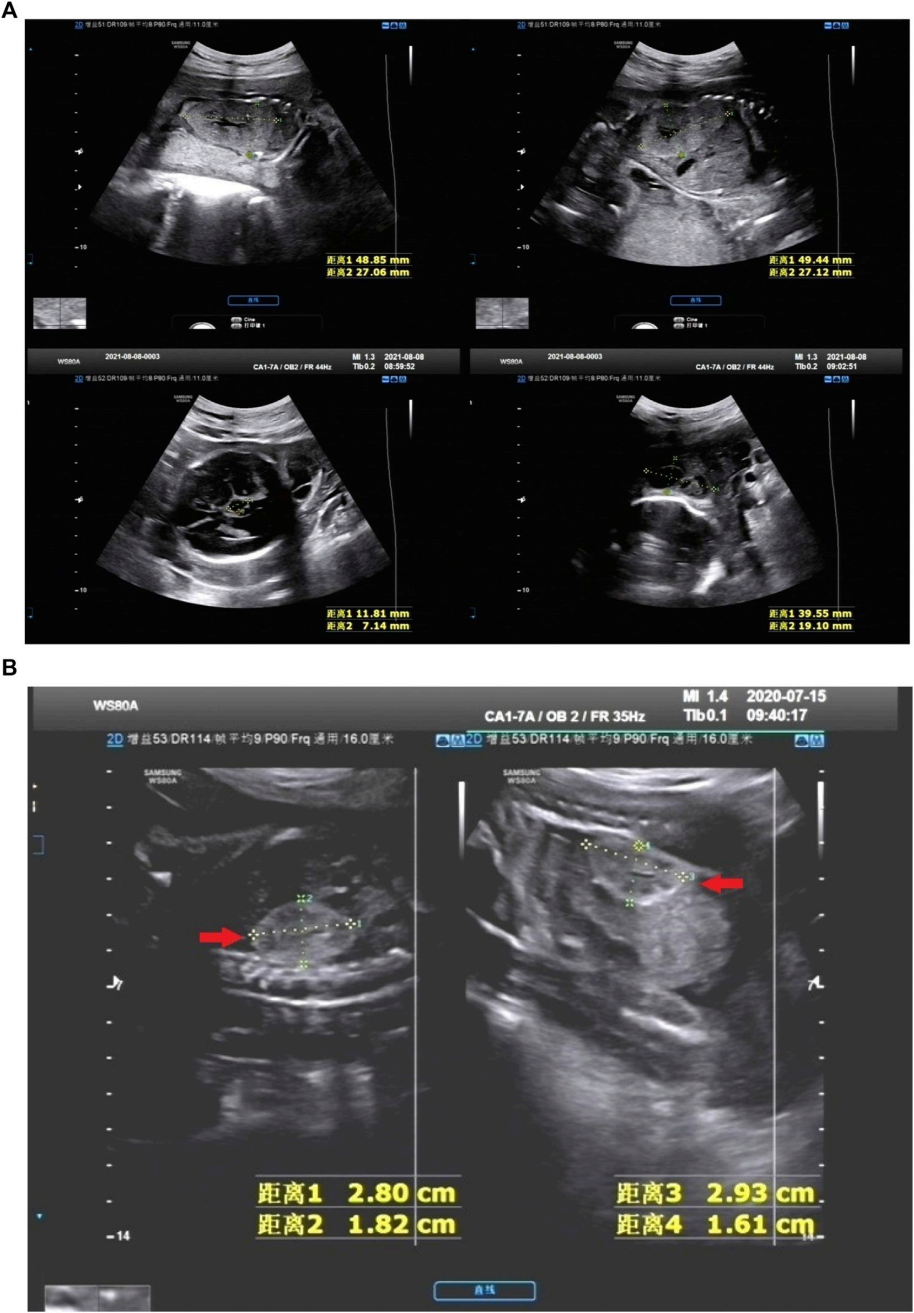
(A) NO. Q210001 in NIHK group, the ultrasound images of 28 weeks showed the size of the left kidney was 48.85 mm*27.06 mm and that of the right kidney was 49.44 mm*27.12 mm, the echo of the renal parenchyma was thickened and enhanced; An 11.81 mm*7.14 mm anechoic area was found in the midline below the third ventricle, Dandy-Walker syndrome with arachnoid cyst was considered; There was a 39.55 mm*19.10 mm anechoic area in the left posterior part of the neck, which was separated by a light band, It was considered as a cystic hygroma of the neck. This case was accompanied by oligohydramnios. (B) NO. 200244 in IHK group, the ultrasound images of 25 weeks showed bilateral hyperechoic kidneys, the size of the right kidney was 28 mm*18 mm and that of the left kidney was 29 mm*16 mm, within normal limits.
